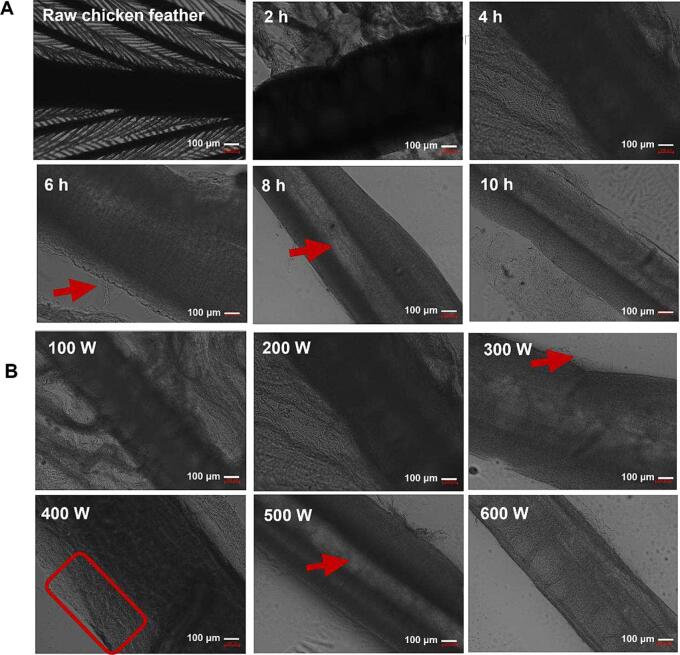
(A) The apparent structure of chicken feather treated without or with ultrasound at 200 W for various time (from 2 to 10 h), and (B) treated with varying ultrasonic power (from 100 to 600 W) for 4 h. The feather were photographed at 2 ∼ 3 cm from the thick end with a magnification of × 100.
